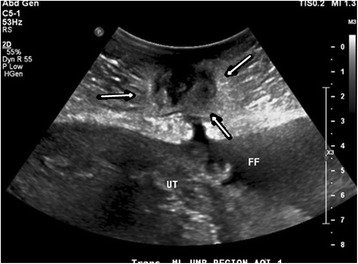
Ultrasound of the abdomen showing free fluid with echoes in the ascitic compartment representing clinically insignificant sound reverberation and floating debris outside the uterine wall. Periumbilical abdomen demonstrates an area of abnormal heterogeneous soft tissue (*arrows*) with an area of complexity posterior to the umbilicus 5.1 × 4.6 × 3.1 cm seemingly with some underlying continuity with the peritoneal cavity. No identifiable abscess or echo suspicious for solid tumor metastasis. *FF* free fluid, *UT* shows the uterine wall
